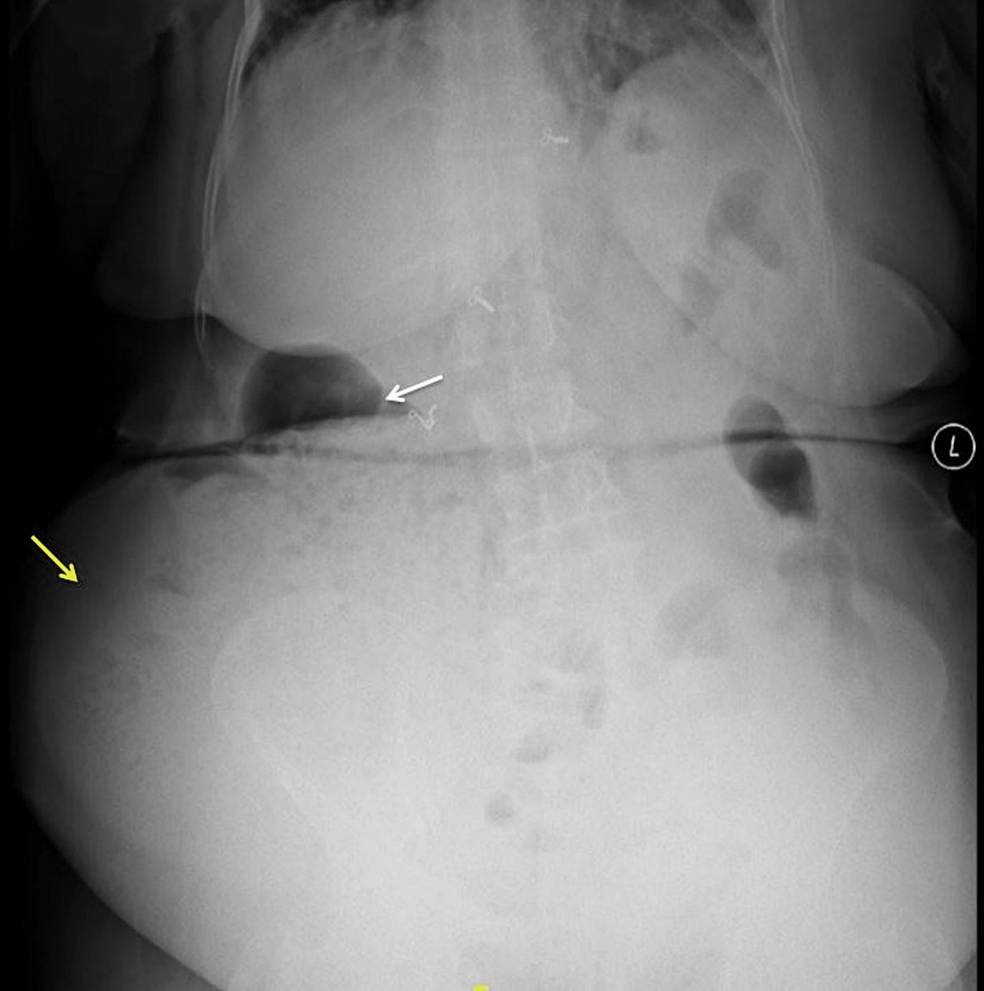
X-ray abdomen (erect view) showing air-fluid level (white arrow) and herniated and obstructed bowel loop (yellow arrow).
